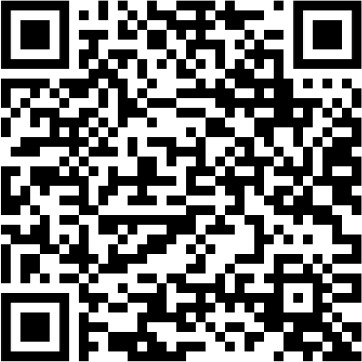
Transesophageal three-dimensional echocardiography clip revealing
